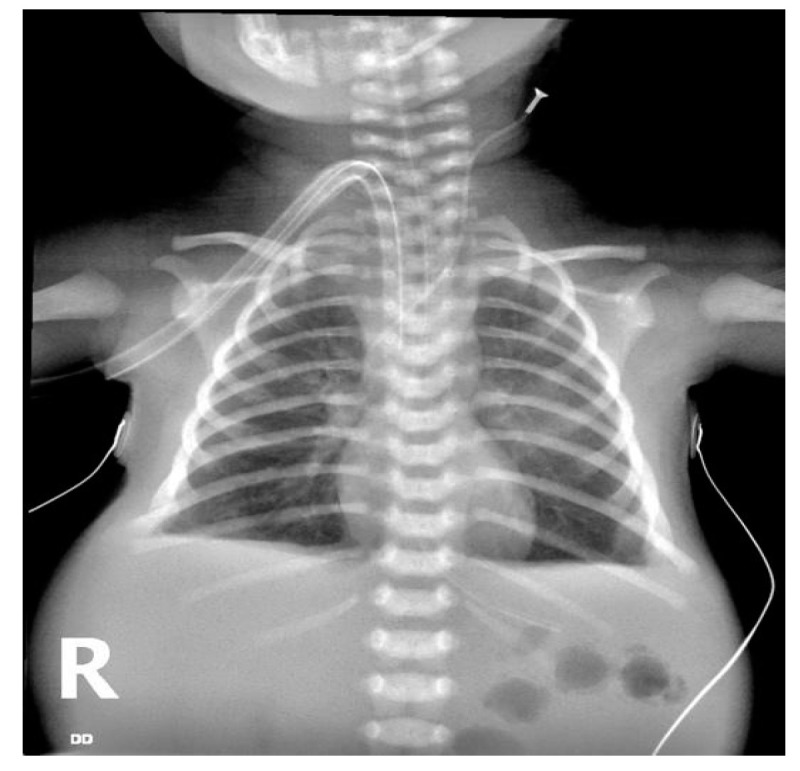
Chest X-ray at birth showing hyperinflated lungs, flattened diaphragm, and enlarged abdomen secondary ascites.
